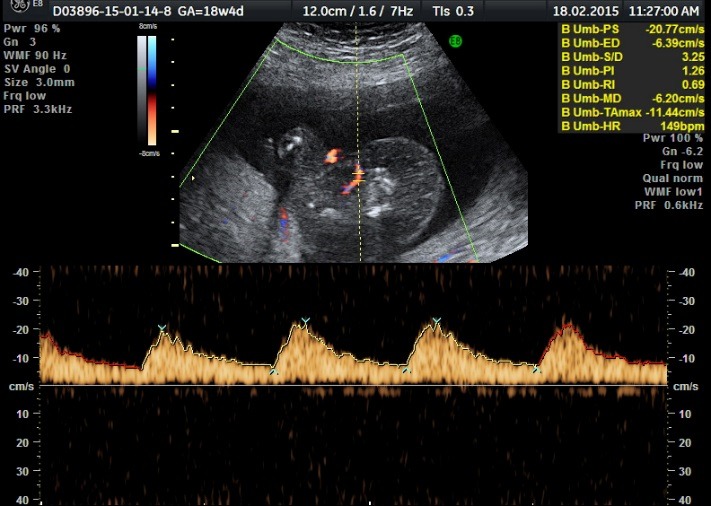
Acardiac twin: Doppler sonography with a high RI index on the umbilical artery
